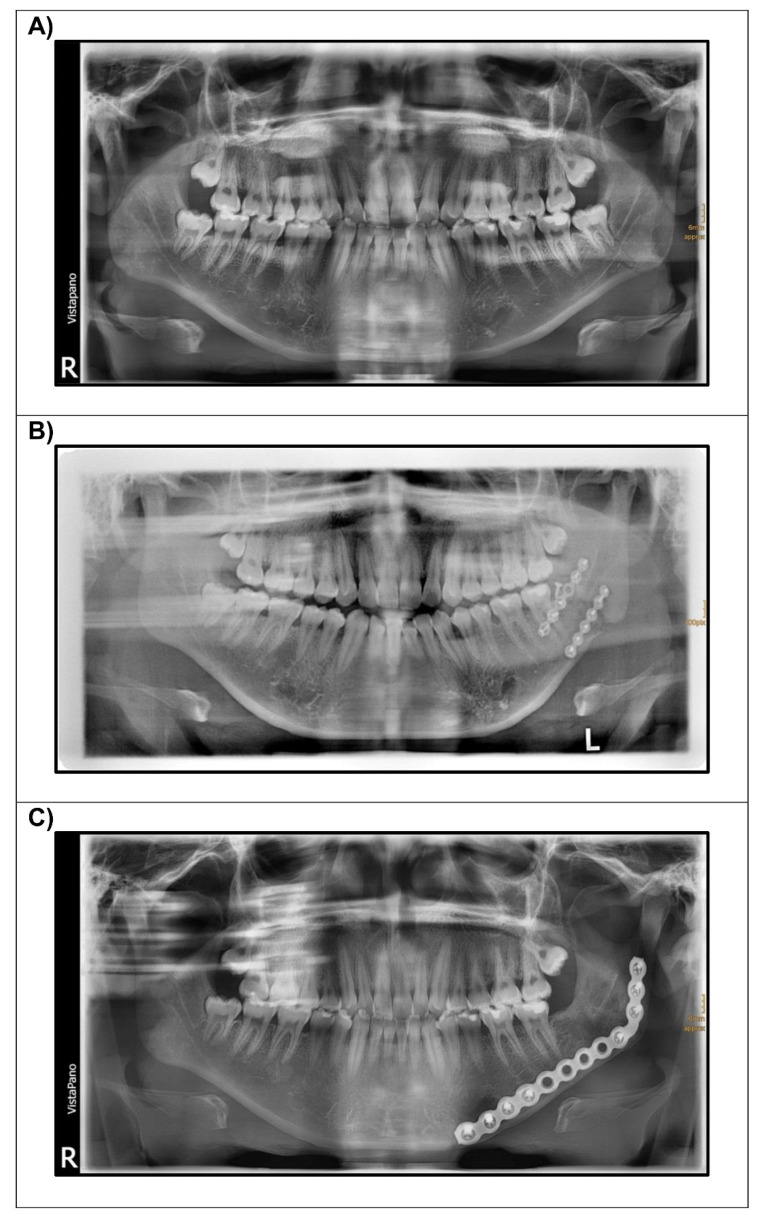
Exemplary patient case of secondary reconstruction using a 2.8 mm reconstruction plate after failure of primary fracture fixation with two miniplates (panoramic radiographs (A) after trauma; (B) after reconstruction with two miniplates; (C) after secondary reconstruction using a reconstruction plate).
